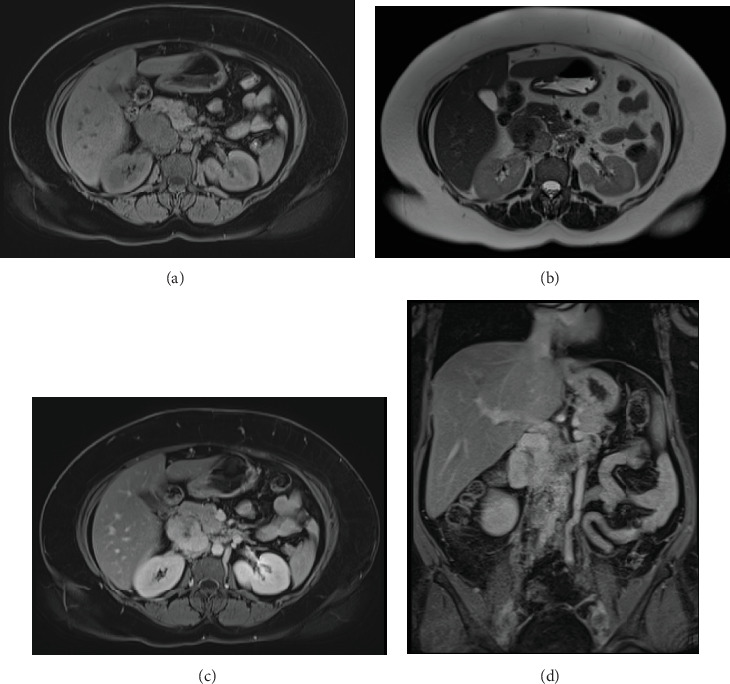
MRI abdomen. Nonenhanced (a) T1 and (b) T2 axial images show an irregular mass within IVC. The mass is best appreciated on postgadolinium-enhanced (c) axial and (d) coronal T1 images, where it appears as a heterogeneously enhancing retroperitoneal mass originating from the IVC with extraluminal extension.
